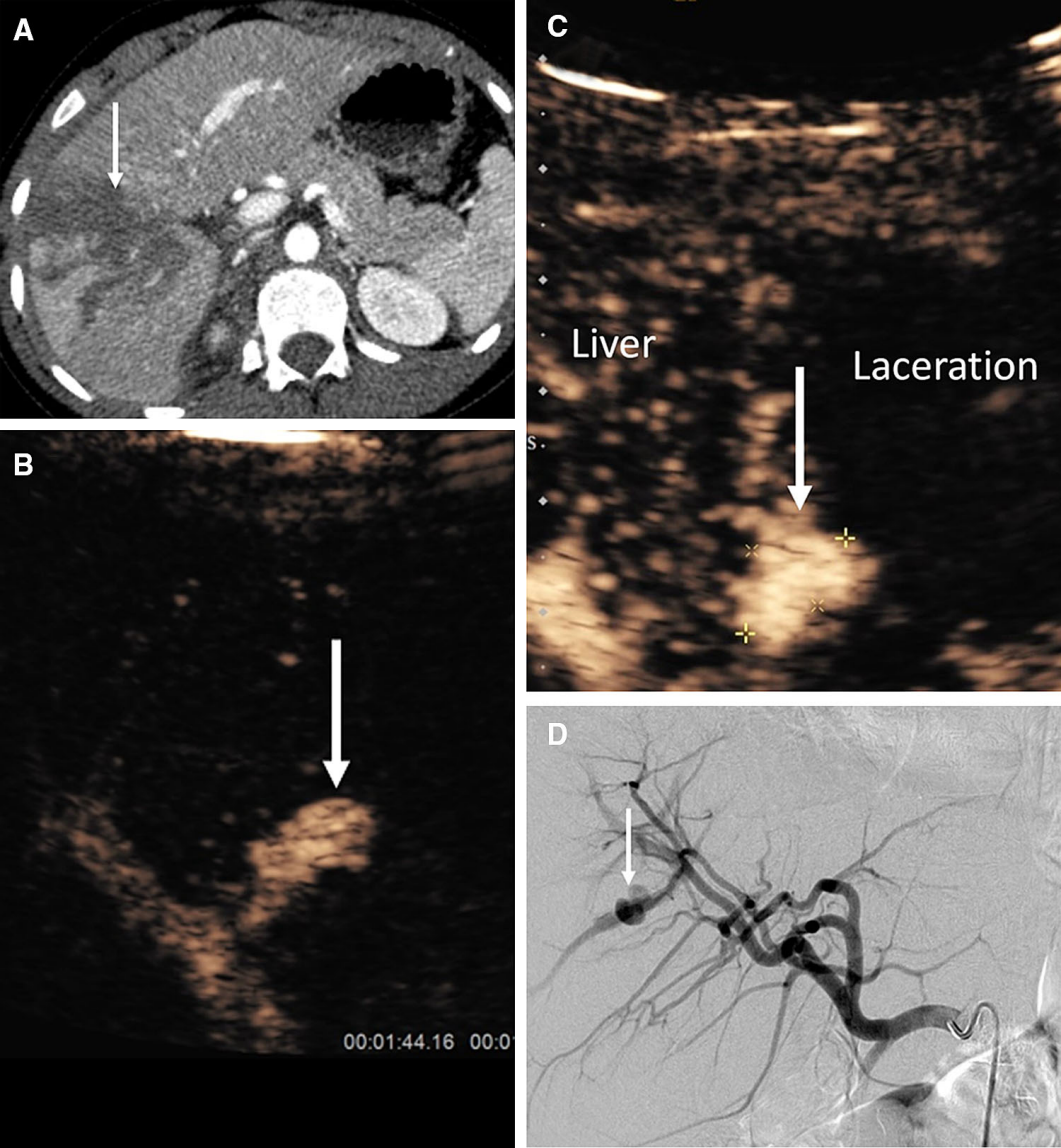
A 14-year-old boy fell downstairs and sustained a grade 4 liver laceration. CECT (axial) shows linear area of liver laceration (A, arrow). CEUS of the liver was performed 5 days post-trauma to evaluate the injury. Sequential CEUS images (B and C) demonstrate a pseudoaneurysm (arrow in B, between cursors and arrow in C). Image B is taken during arterial phase akin to the CT angiographic phase with microbubble contrast only seen in the hepatic artery and pseudoaneurysm. The later image C shows the presence of microbubble contrast within the liver. The part of the liver which lacks microbubble contrast correspond to area of liver laceration. The pseudoaneurysm was confirmed during conventional hepatic artery catheter angiography (D, arrow) and subsequently successfully embolized with vascular coils
